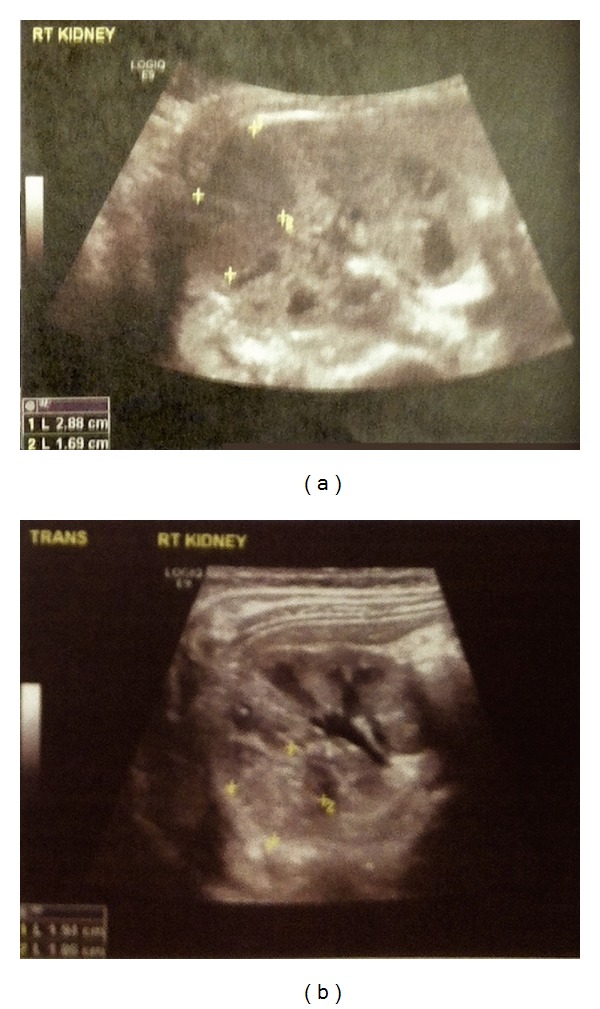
(a) Abdominal ultrasound showing an enlarged right kidney with a large hypoechoic region with no corticomedullary differentiation before chemotherapy treatment; (b) decrease of right kidney large hypoechoic lesion dimensions after 4 months of treatment.
